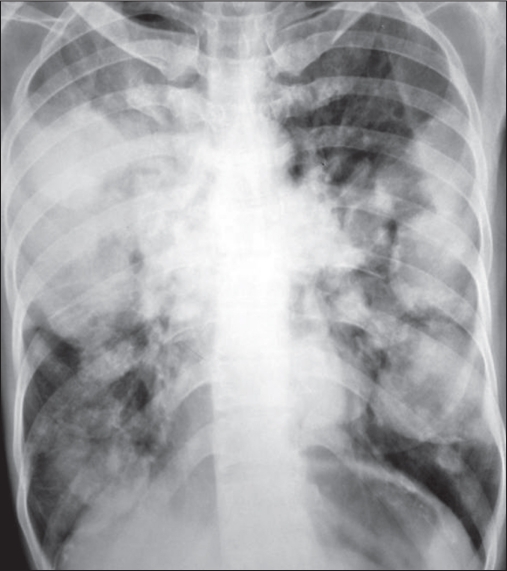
Chest X-ray PA view multiple rounded homogenous parenchymal shadows of varying size, 2–5 cm in diameter in both lung fields. Some of these shadows coalesce with each other and surrounding mediastinal structures
